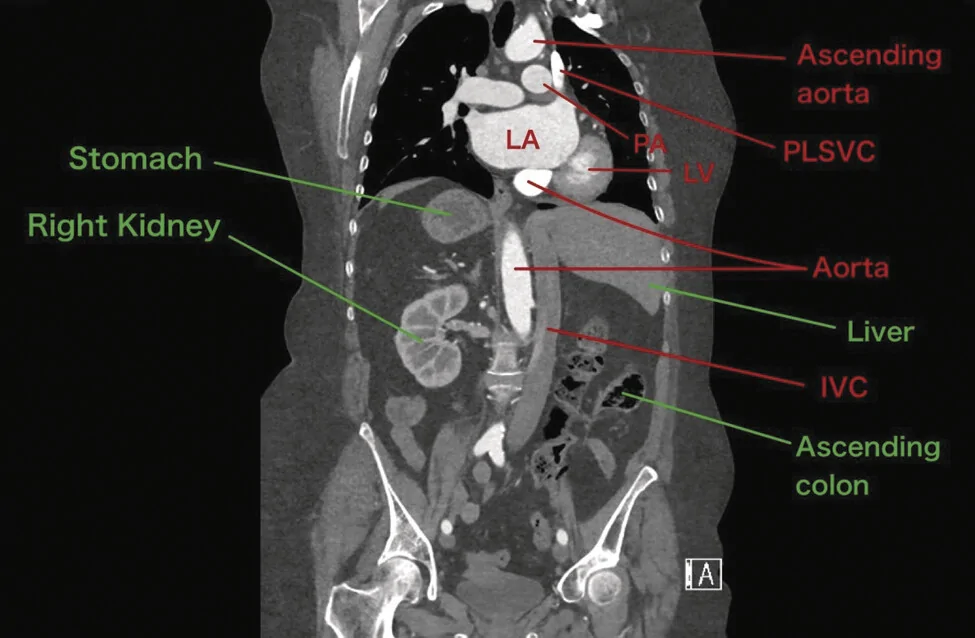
Heterotaxy and systemic venous anatomy (coronal view). Coronal contrast-enhanced computed tomography demonstrating heterotaxy with a mirror-image arrangement of the thoracoabdominal organs. Note the left-sided IVC and right-sided abdominal aorta, confirming the reversal of normal lateralization. A PLSVC is also visualized draining into a dilated coronary sinus. IVC, inferior vena cava; LA, left atrium; LV, left ventricle; PA, pulmonary artery; PLSVC, persistent left superior vena cava.
